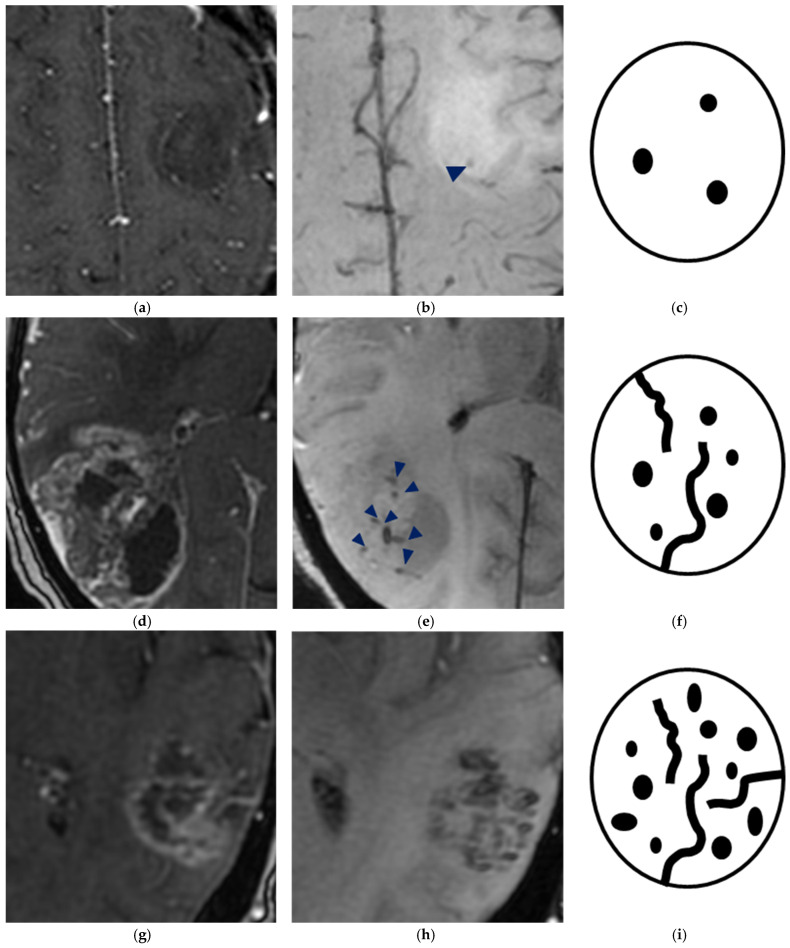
Semiquantitative ITSS assessment: (a–c) grade 1: 1–5 dot-like or linear ITSS (arrowhead); (d–f) grade 2: 6–10 dot-like or linear ITSS (arrowheads); and (g–i) grade 3: ≥11 dot-like or linear ITSS. MRI sequences: postcontrast 3D T1 BRAVO (a,d,g); 3D SWAN (b,e,h).
